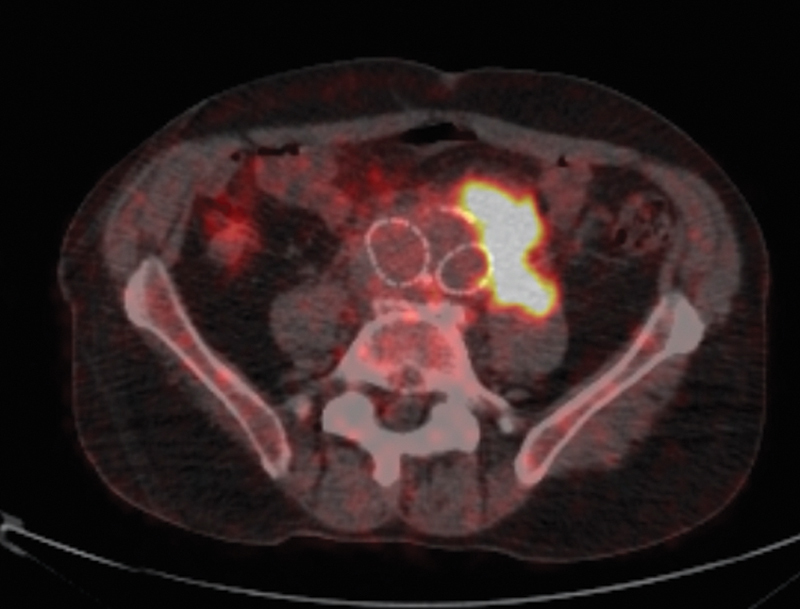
Axial view of the positron emission tomography-computed tomography showing a high uptake extending into the left iliac fossa.
